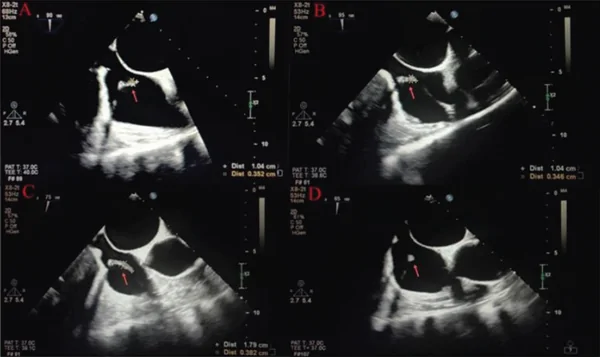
Serial transesophageal echocardiography (TEE) images of the right atrial lead thrombus. (A) Initial TEE image prior to admission showing a 10 × 3.5 mm mobile, hyperechoic mass attached to the right atrial lead, suggestive of thrombus. (B) One month after initiation of rivaroxaban therapy, the thrombus remained unchanged in morphology and size. (C) Three months after switching to warfarin (INR 2.0–3.0), the thrombus had enlarged to 18 × 3.8 mm, indicating inadequate anticoagulant effect. (D) Six months after INR was adjusted to 2.5–3.0, complete resolution of the thrombus was achieved.
